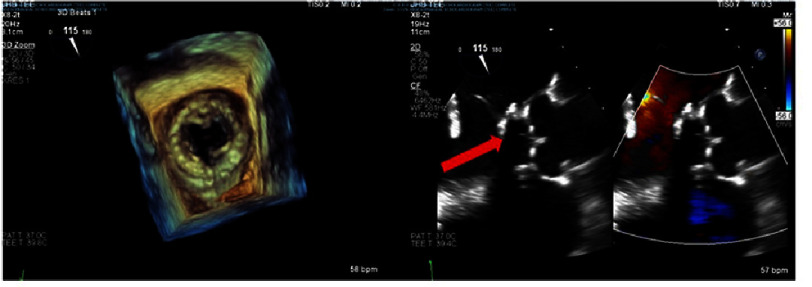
Preoperative transesophageal echocardiography. Left: Three-dimensional reconstruction of the previously placed, normally functioning bioprosthetic mitral valve with LVOT obstruction caused by a strut. Right: Mid-esophageal aortic long-axis view demonstrates obstruction of the LVOT by a strut (arrow). LVOT; left ventricular outflow tract.
